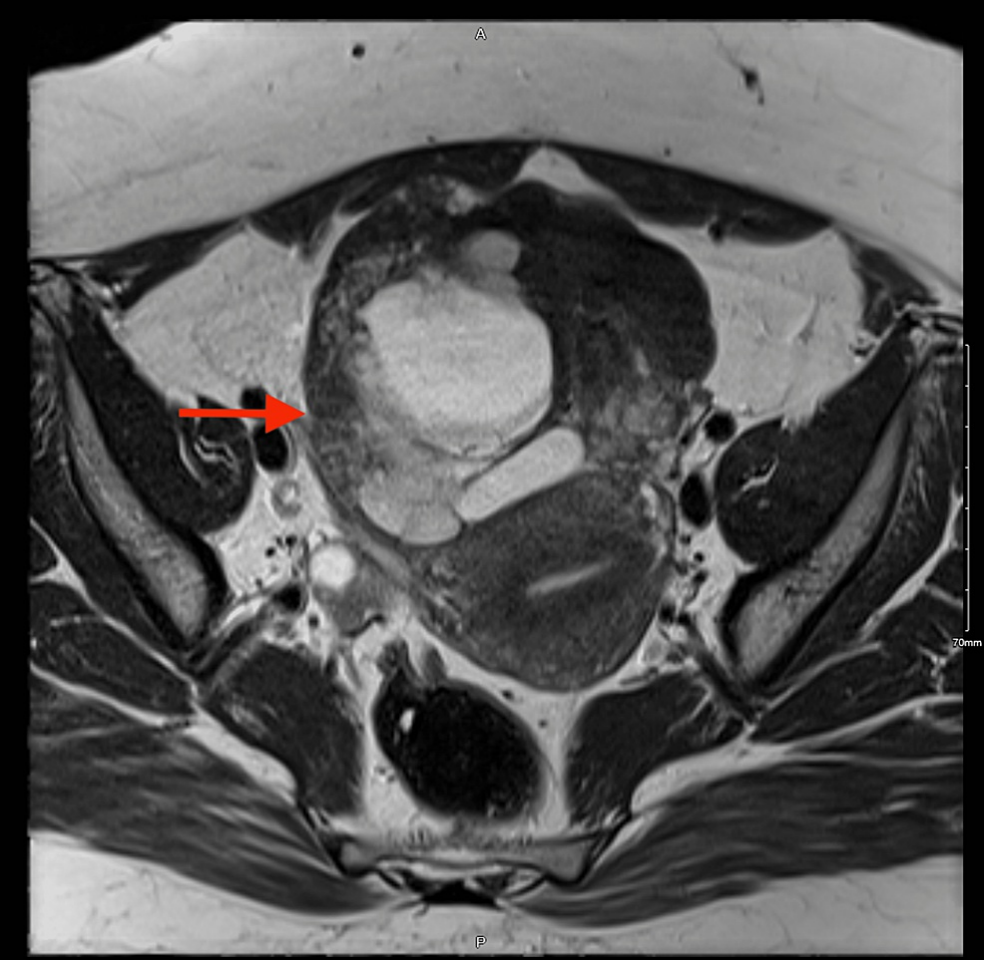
Magnetic resonance imaging (MRI) axial view. A large multiseptated cystic lesion is seen in the anterior central aspect of the pelvis located anterior to and abutting the uterus, capping and inseparable from the left ovary and extending anteriorly to the posterior aspect of the right rectus abdominis muscle. Laterally, the lesion is bounded on the left side by the stretched right round ligament; posteriorly, it is resting upon the urinary bladder dome and causing mild compression.
